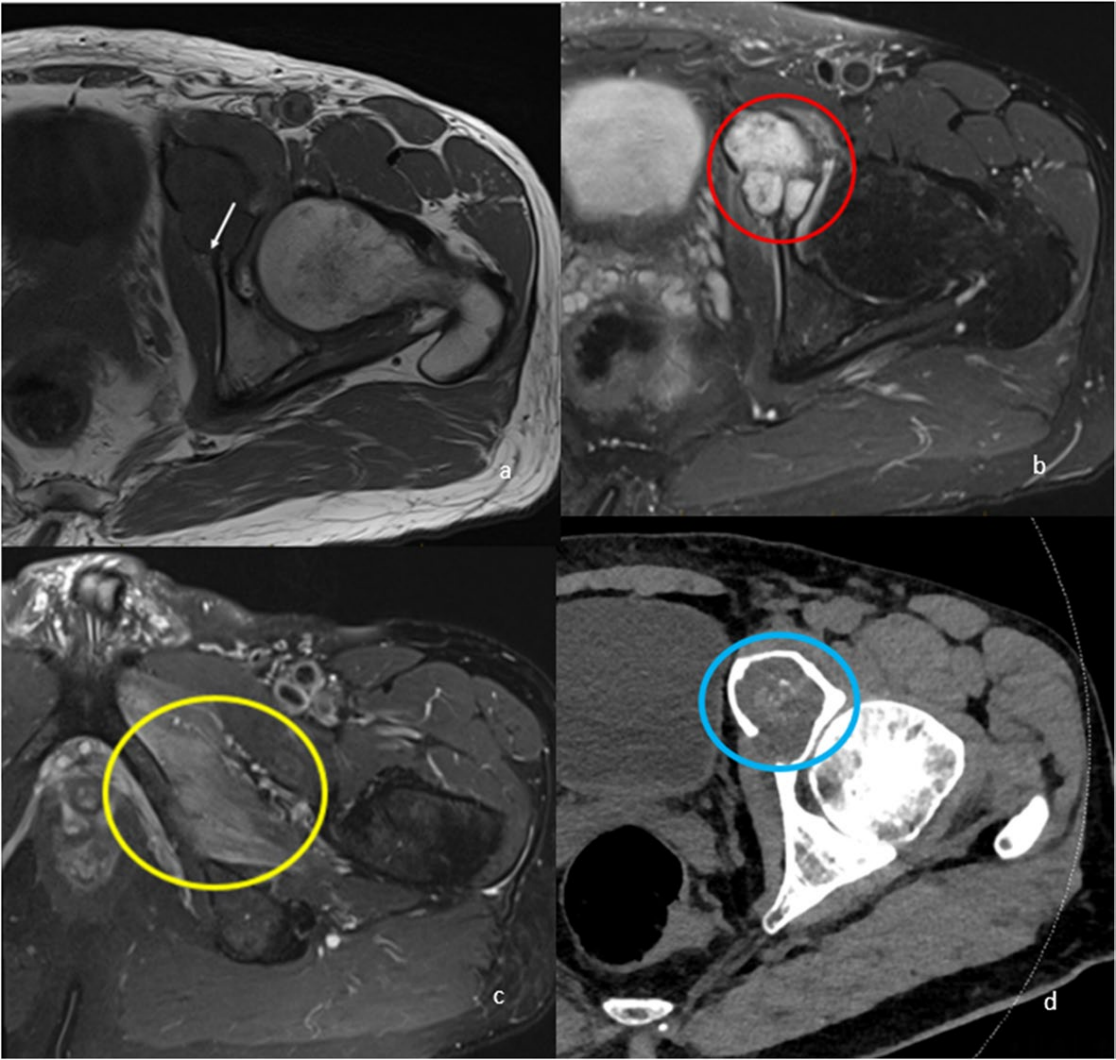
61-year-old male with chondrosarcoma in the left superior pubic ramus and anterior acetabulum. Axial T1 (a) and PDFS (b, c) sequences demonstrate the fluid-hyperintense intramedullary lesion with chondroid calcification (red circle). There is extraosseous extension into the left obturator foramen/canal, encroaching on the left obturator nerve (white arrow), resulting in nerve entrapment and secondary denervation oedema of the obturator externus, adductor brevis and magnus (yellow circle). CT reveals chondroid calcification of the tumour (blue circle) (d)
